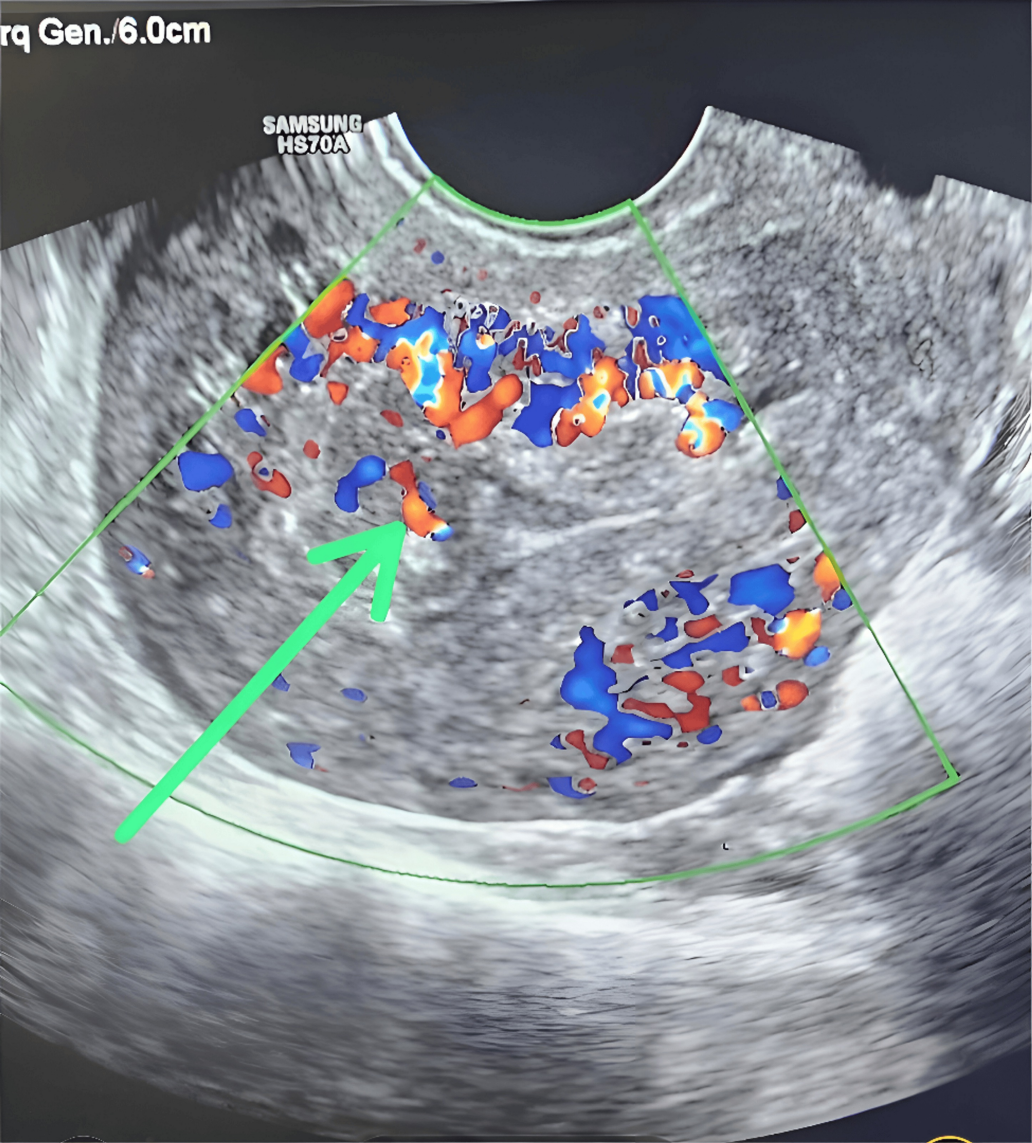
Transvaginal color Doppler ultrasound demonstrating marked irregular and thickened heterogeneous material within the uterine cavity, as shown by the green arrow, with prominent internal vascularity
